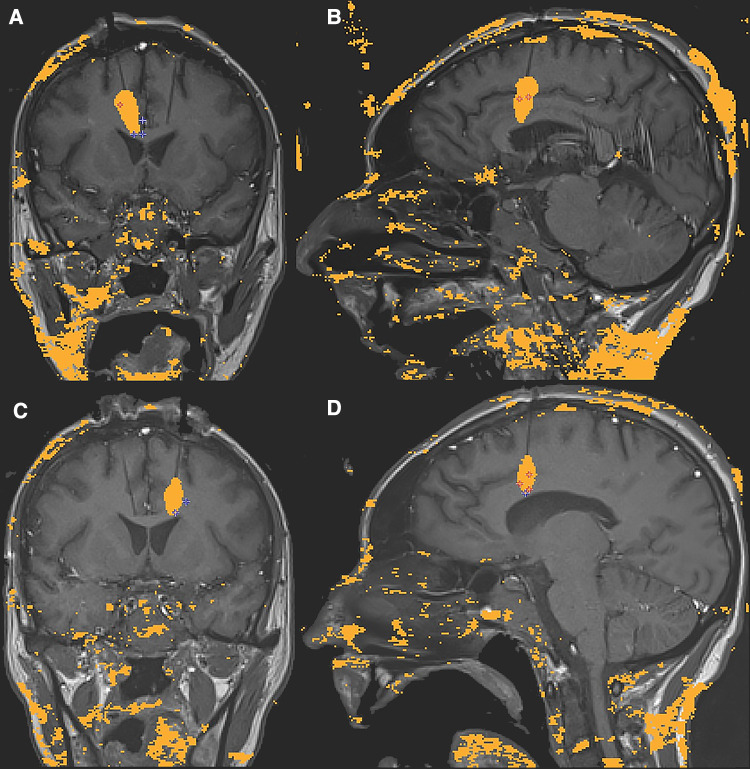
Intraoperative sagittal and coronal MRI thermometry images of the completed right (A,B) and left (C,D) anterior cingulotomy laser-induced thermal lesions.
